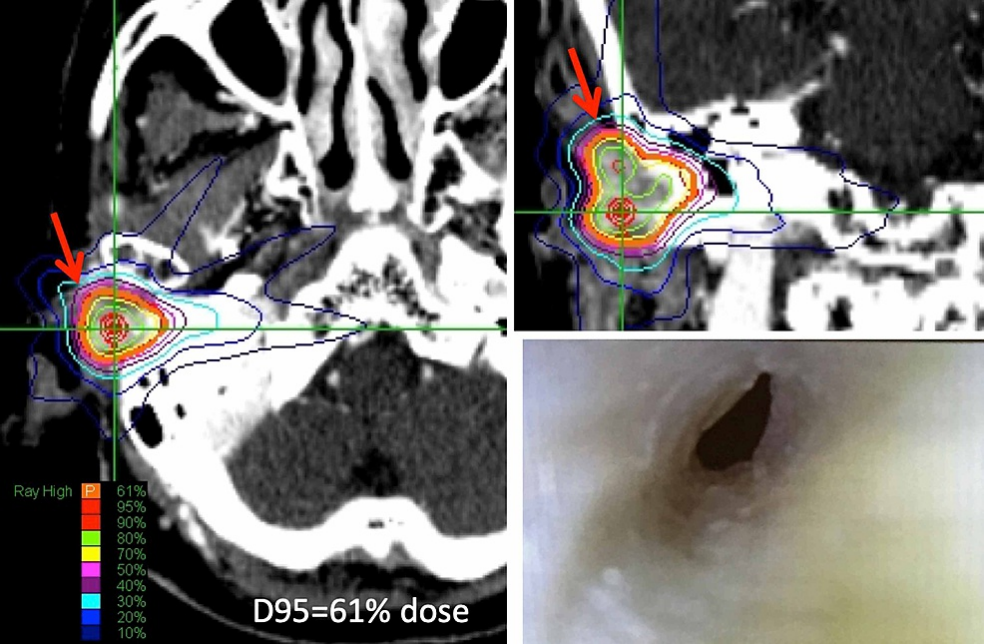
Dose Planning for Case 3 and Follow-up Axial (left) and coronal (right upper) images of iodine enhancement CT on MultiPlan workstation showed a small external auditory canal tumor (arrow). A prescription dose of D95%=30 Gy/ 5 fractions was adopted. Complete remission was obtained and maintained until 19 months after the treatment. Otoscopy (right lower) revealed patency of the external auditory canal 19 months after the treatment.
